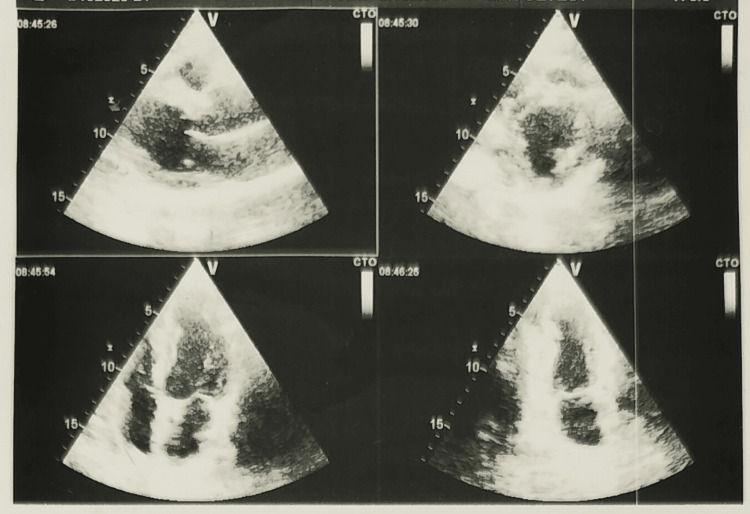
Echocardiogram shows that the distal mid-anteroseptal wall of the left ventricle is hypokinetic. Apical interventricular septum, mid apical anterior and inferior walls are hypokinetic. Mild to moderate left ventricular systolic dysfunction (ejection fraction 43%)
